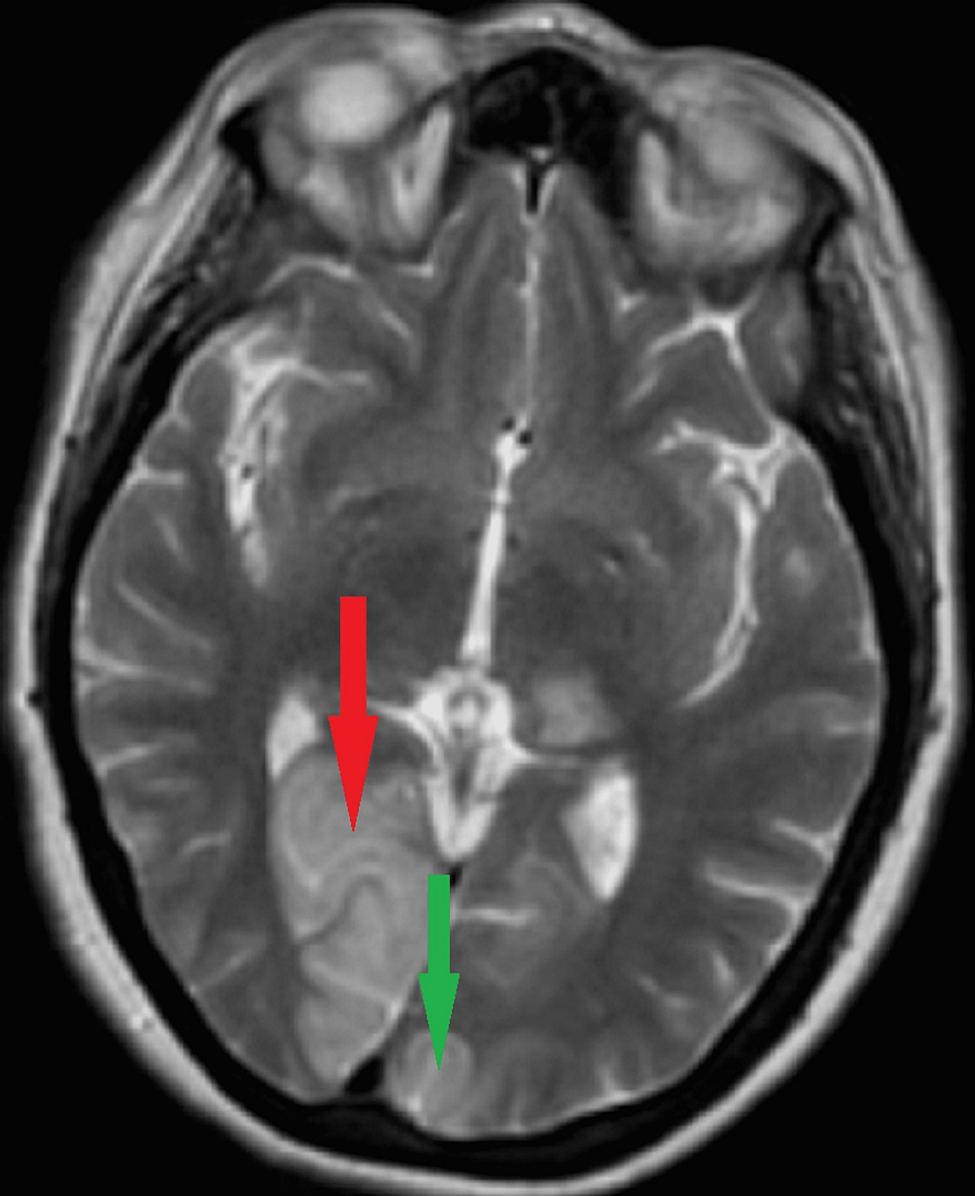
Representative T2 view of the MRI of the brain showing areas of infarction in bilateral PCA stroke Axial view of the MRI of the brain. The areas of infarction are indicated as follows: red arrow indicates right occipital lobe, while green arrow indicates left occipital lobe.
